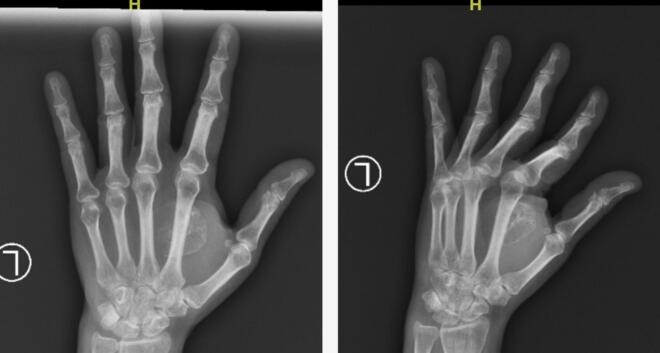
Anteroposterior and lateral view of hand X-ray showing a soft tissue mass with internal calcifications.
